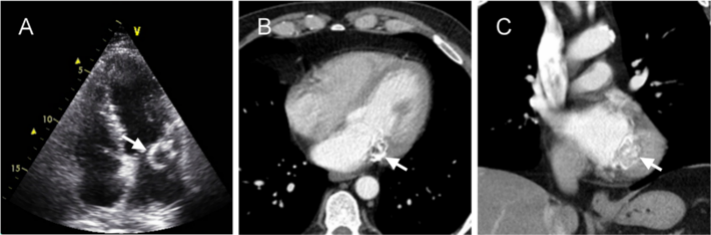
2D echocardiography and multi-slice CT findings. Echocardiography (A) showed an echolucent mass with a highly echogenic rim (arrow) attached to the mitral valve. Transverse (B) and coronal (C) chest CT images showed a mass (arrows) adhering to the posterior mitral valve leaflet with peripheral calcification.
